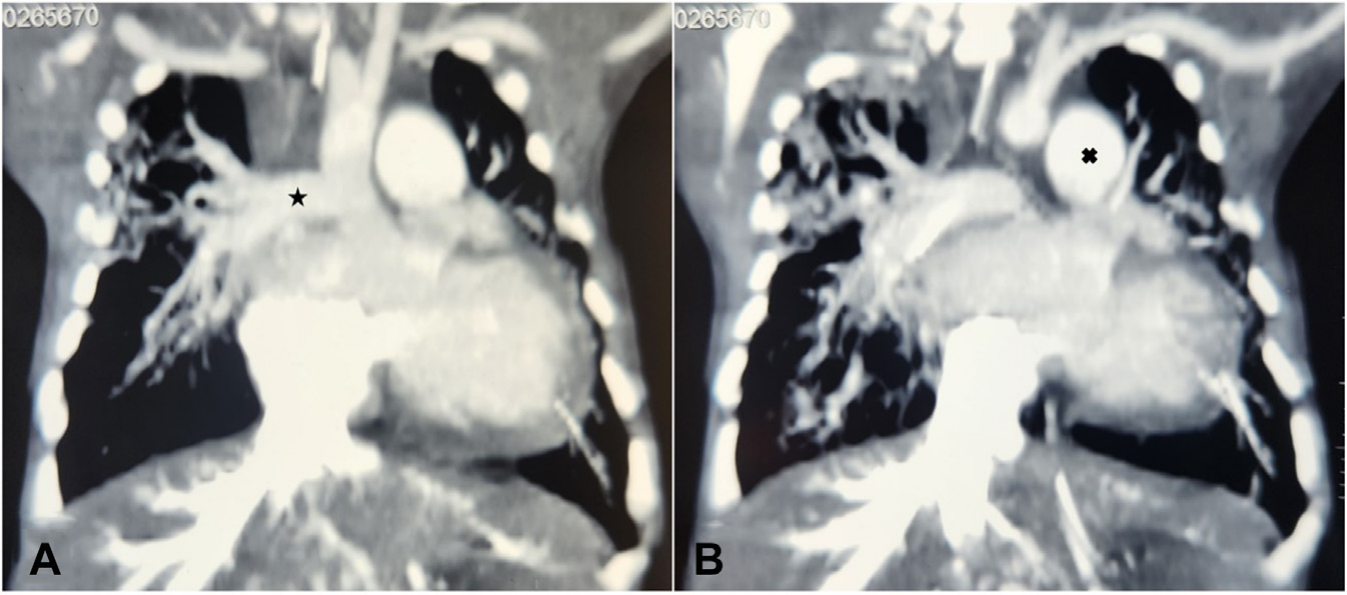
A, Contrast-enhanced computed tomography image in coronal section showing abnormal origin of the RPA from the ascending aorta. B, Contrast-enhanced computed tomography image in coronal section showing main pulmonary artery continuing as left pulmonary artery.
